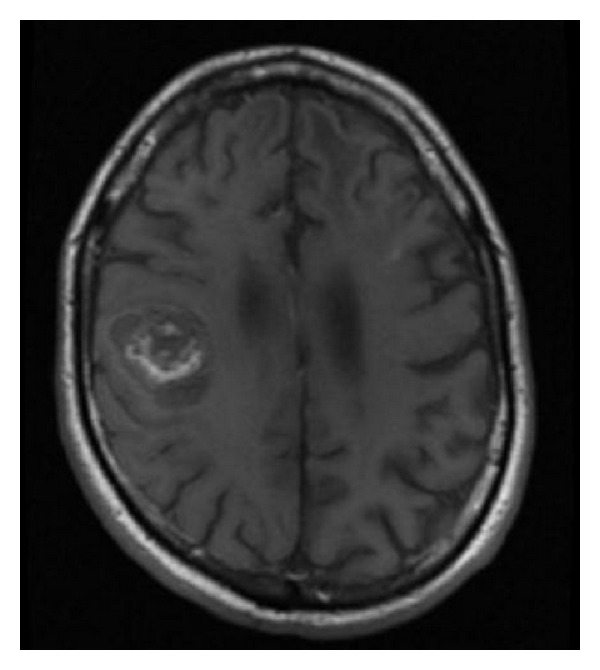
T1-postcontrast MRI showing a 2.7 × 3.5 × 3.1 cm hemorrhagic mass involving the right precentral gyrus. Following surgical resection, pathology confirmed metastatic melanoma.
